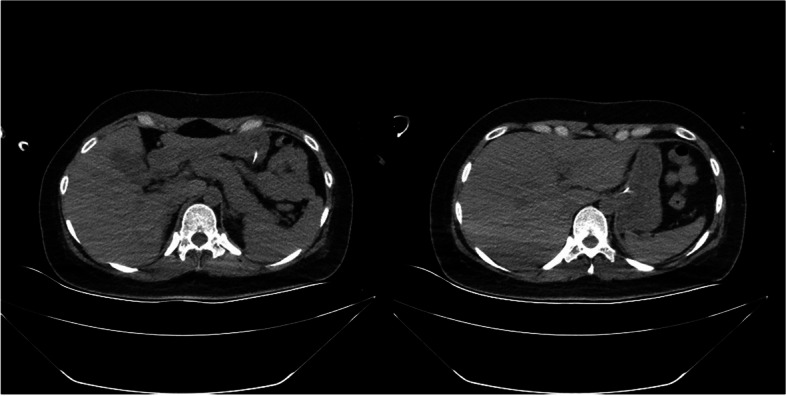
Abdominal CT on the fifth day of admission.Both the liver and spleen were normal in size and shape
